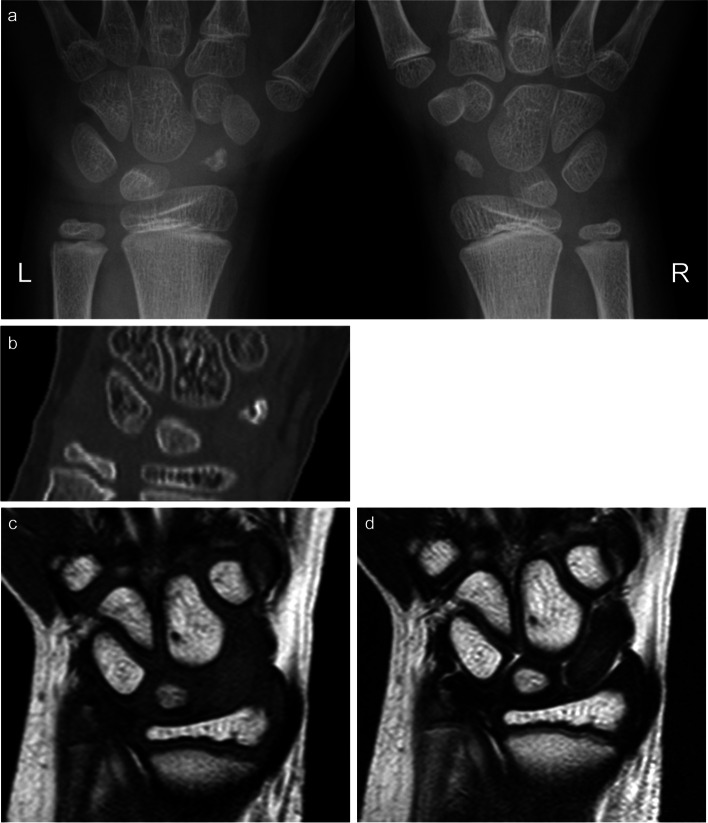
Radiologic image findings at the initial visit. Plain radiography showed osteosclerosis (a) and CT revealed segmentation of left scaphoid bone (b). Both MRI T1- and MRI T2-weighted images showed low intensity on the whole scaphoid bone, which meant osteonecrosis (c, d)
